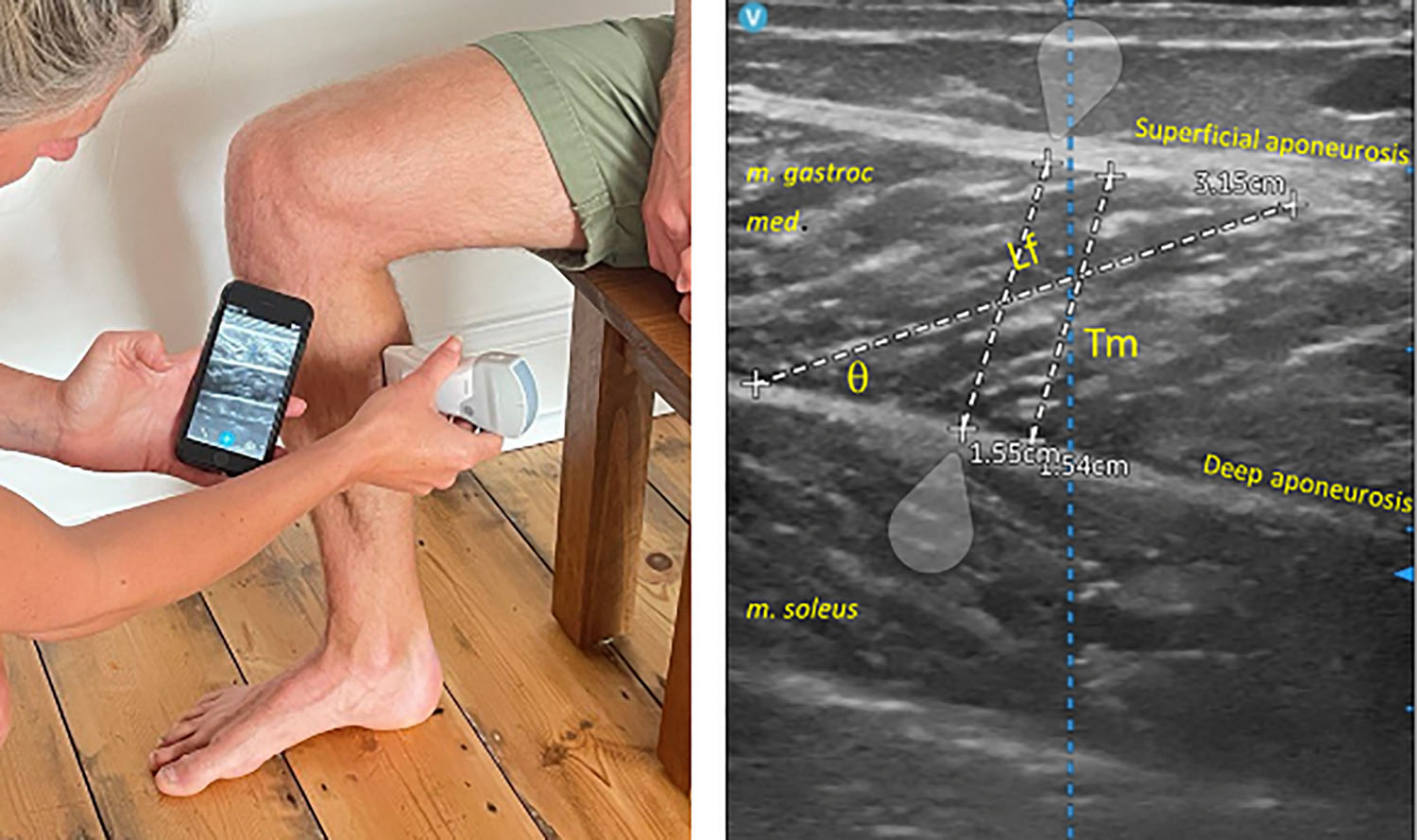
Ultrasound scan of calf in longitudinal plane (a) and muscle architectural measurements (b).
